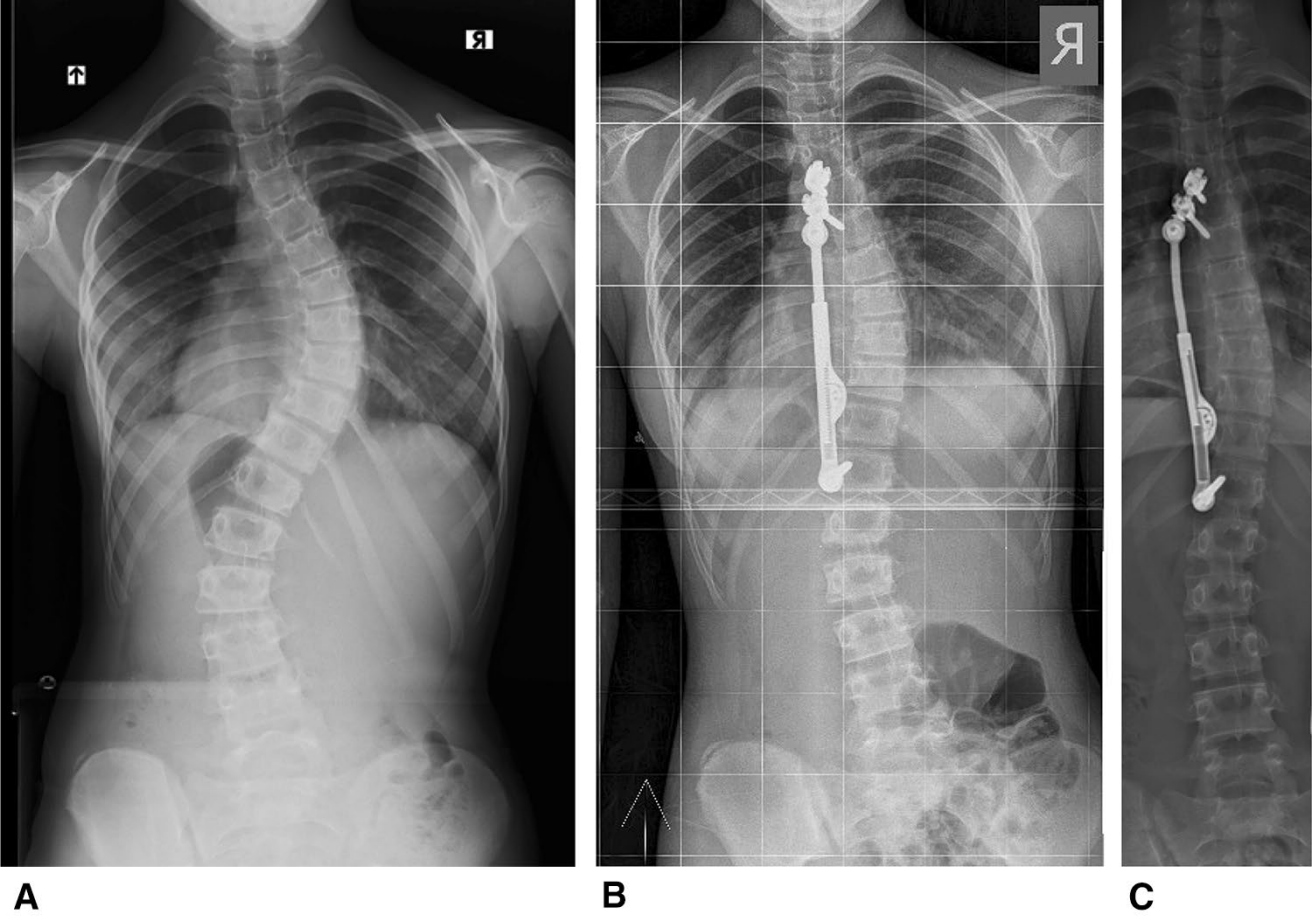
a 12-year old girl Lenke 1 58°, Risser grade 1. T9 coronal wedging is 10°. b Immediate postoperative x-ray. c At 2 years follow-up the curve measures 20° and coronal wedging is 4° (Risser 4 stage). In this case the device was anchored to the spine by two proximal pedicle screws via an “extender”
